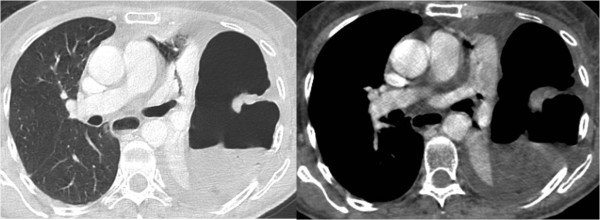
CT scan at sub-carinal region shows air fluid level above diaphragm, indirect sign of a bowel herniation. Moderate dilation of esophagus is visible (arrow) with fluid stagnation in the lumen. Partial collapse of left lower lobe and fluid in the pleural space is seen.
